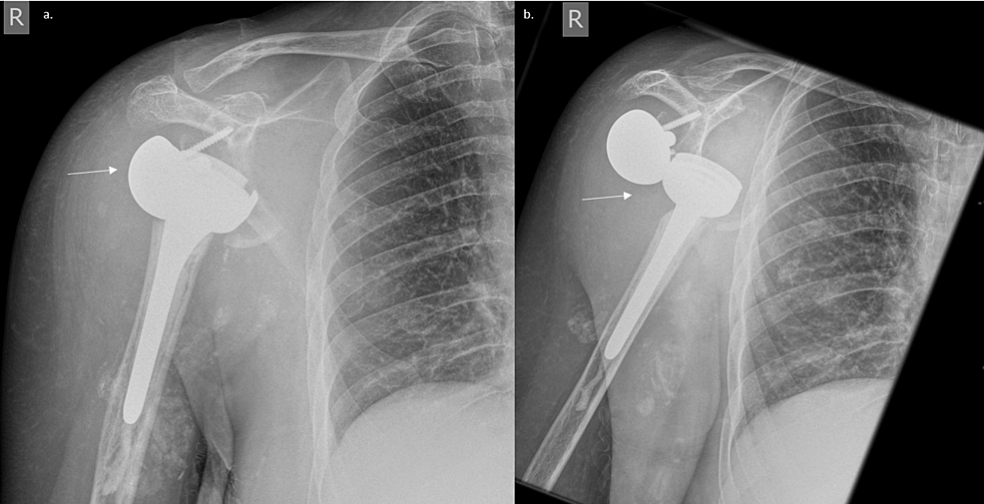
Radiograph of shoulder post-reverse total shoulder arthroplasties (TSA) a. AP of the prosthesis b. PA of the prosthesis The shoulder x-ray shows the dislocation of the reverse arthroplasty
